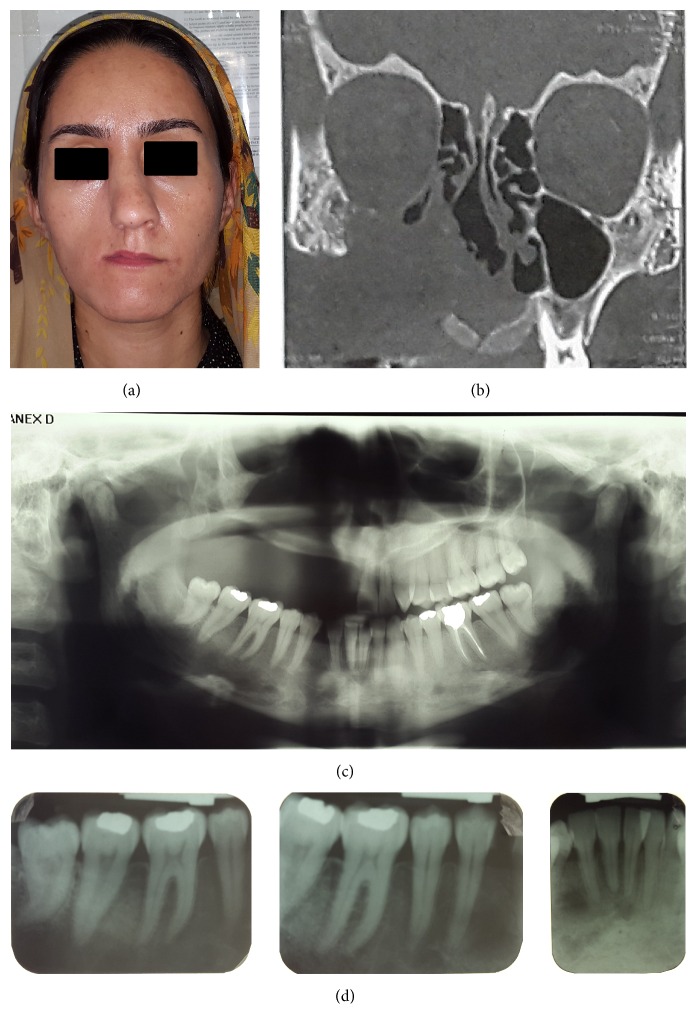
Postoperative course (18 months after surgery). (a) Extraoral frontal photography. (b) CT scan coronal axis. (c) Panoramic view. (d) Periapical views.
